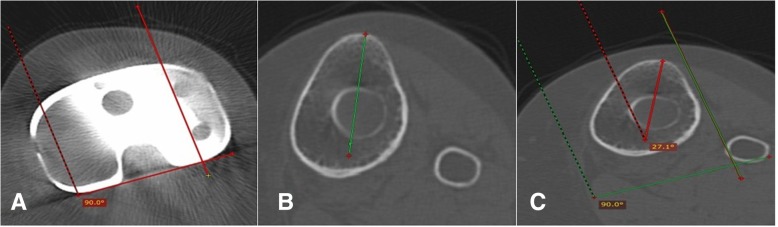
a Axial computed tomography (CT) image through the tibial component polyethylene showing the tibial component axis, which is defined as the perpendicular to the posterior margin of the component. b Axial CT image through the tibial tuberosity showing the tibial tuberosity axis (T.T.A), which is defined by the line connecting the geometric center of the tibial plateau to the tip of the tibial tubercle. c The T.T.A from b is superimposed on the tibial component axis (T.C.A) from a. The rotation of the tibial component is recorded as the angle subtended by the T.T,A and the T.C.A
